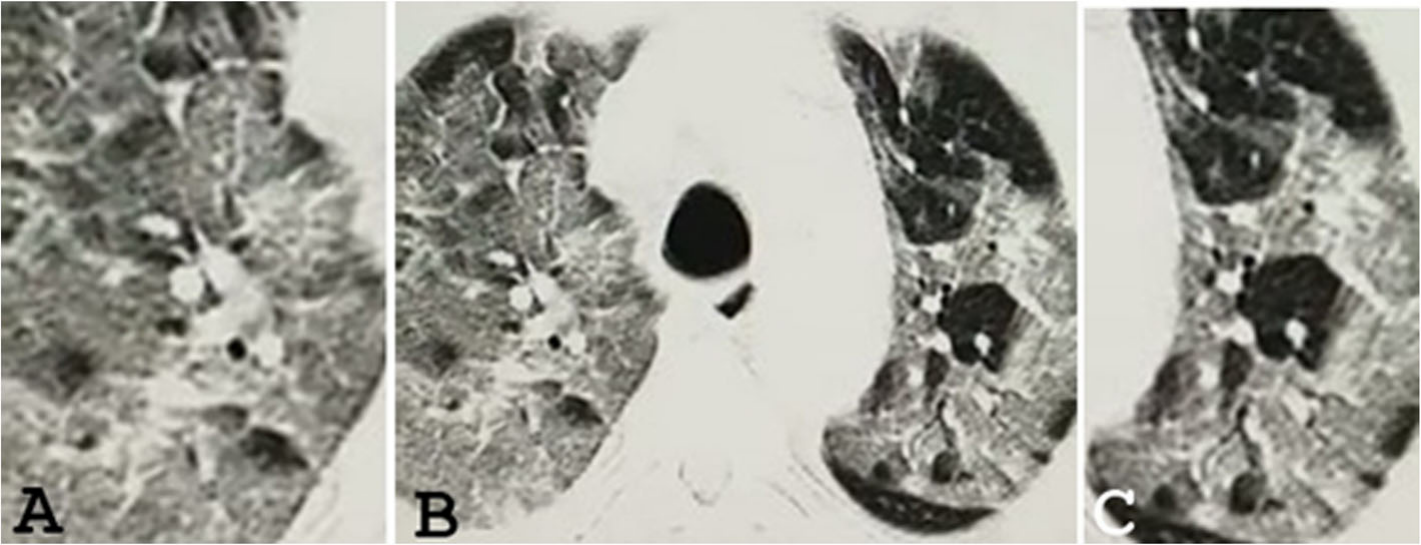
An axial CT image in a 73-year-old patient with COVID-19 infection 10 days after onset of dyspnea. a and c are magnified views highlighting the diffuse ground-glass opacity with crazy paving appearance. b An axial CT image shows diffuse ground-glass opacity with crazy paving appearance
